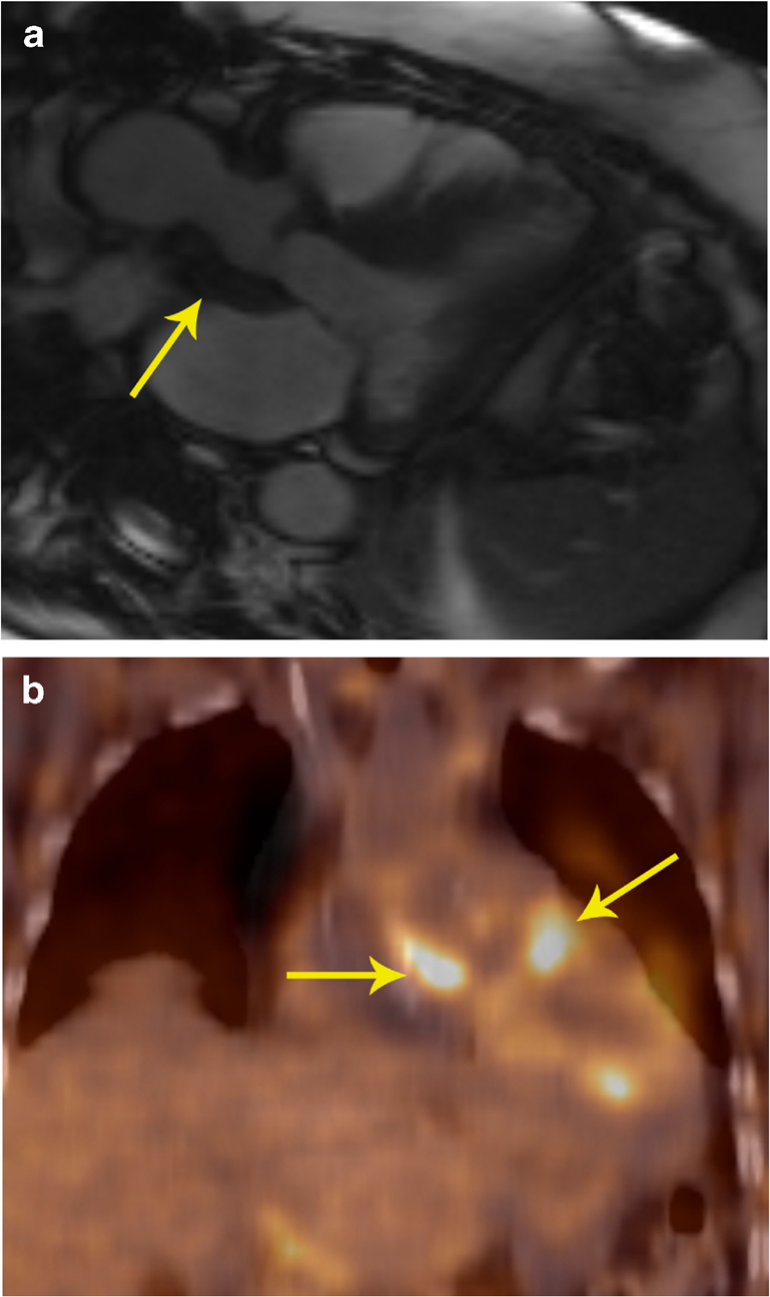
Perivalvular abscess. a Three-chamber Cine SSFP MRI image in a patient with history of bioprosthetic aortic valve replacement who presented with acute onset chest pain shows a soft-tissue intensity lesion surrounding the aortic valve and the aortic root (arrow). Contrast was not administered due to severe renal dysfunction. b Coronal FDG PET/CT in the same patient shows intense uptake along the lesion, which indicates that this is active inflammation/infection. Follow up MRI obtained two days later showed liquefaction and formation of abscess. This was treated surgically
